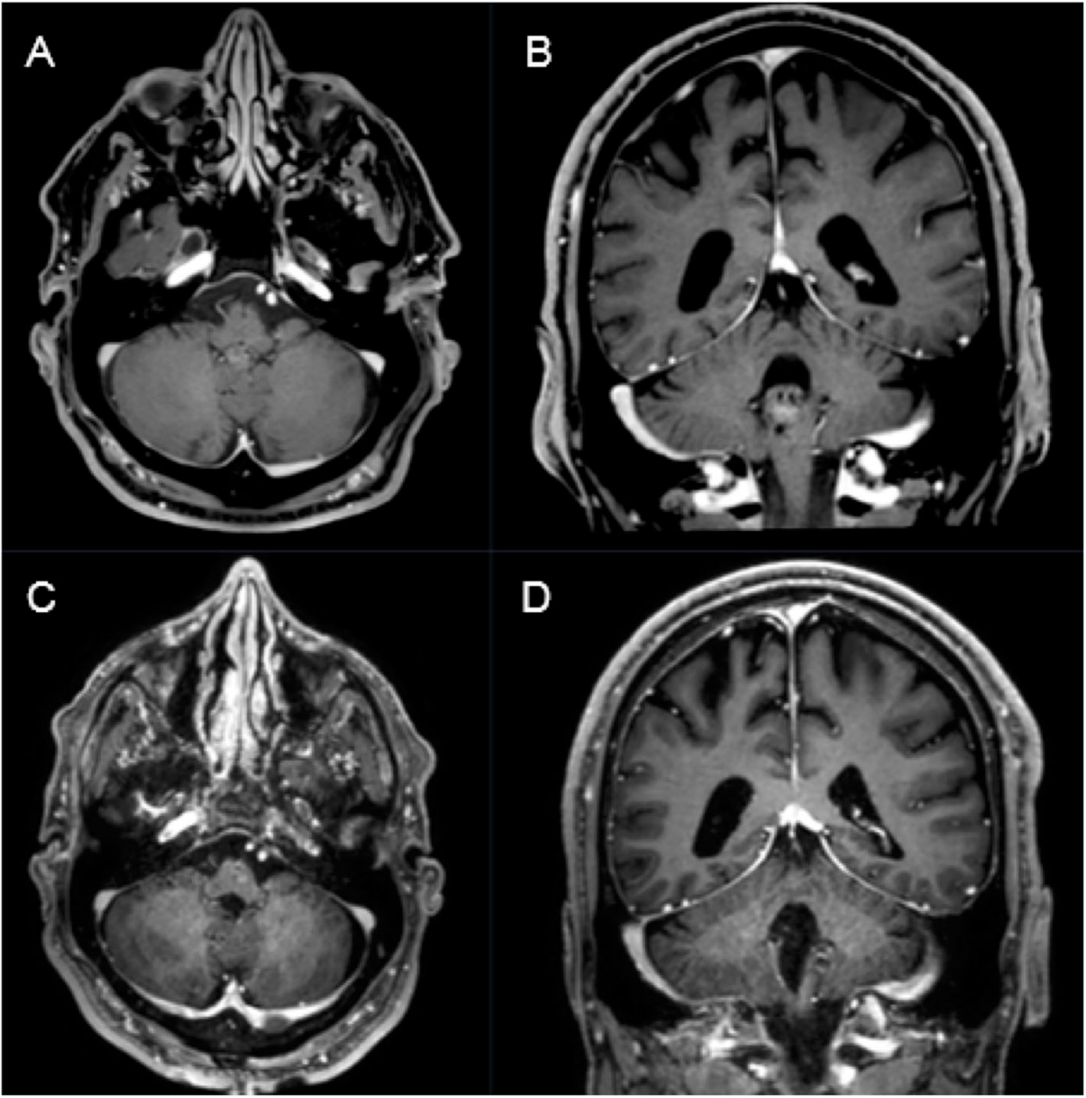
A 27-year-old female patient presented with slight headache and intermitting vertigo. Preoperative T1-weighted gadolinium enhanced MRI showing a heterogeneously enhancing intraventricular mass on the ground of the fourth ventricle consistent with an ependymoma (a,b). Postoperative T1-weighted gadolinium enhanced MRI showing complete removal of the tumor through a median suboccipital telovelar approach (c,d). Pathological findings confirmed WHO grade II ependymoma
